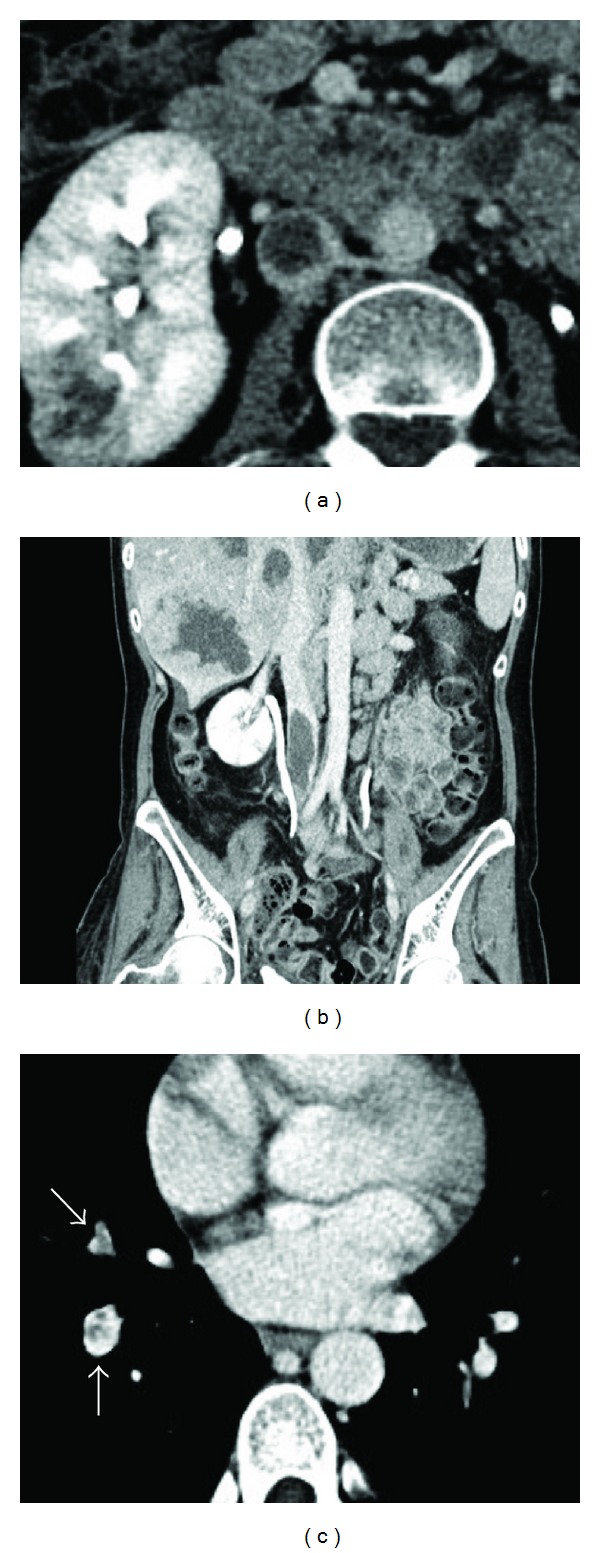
Contrast-enhanced abdominal ((a) and (b)) and chest (c) computed tomography. A massive, floating thrombus (approximately 18 × 20 mm) extended from the right common iliac vein to the infrarenal inferior vena cava; an intra-arterial thrombus occluded a right lower lobe pulmonary artery.
